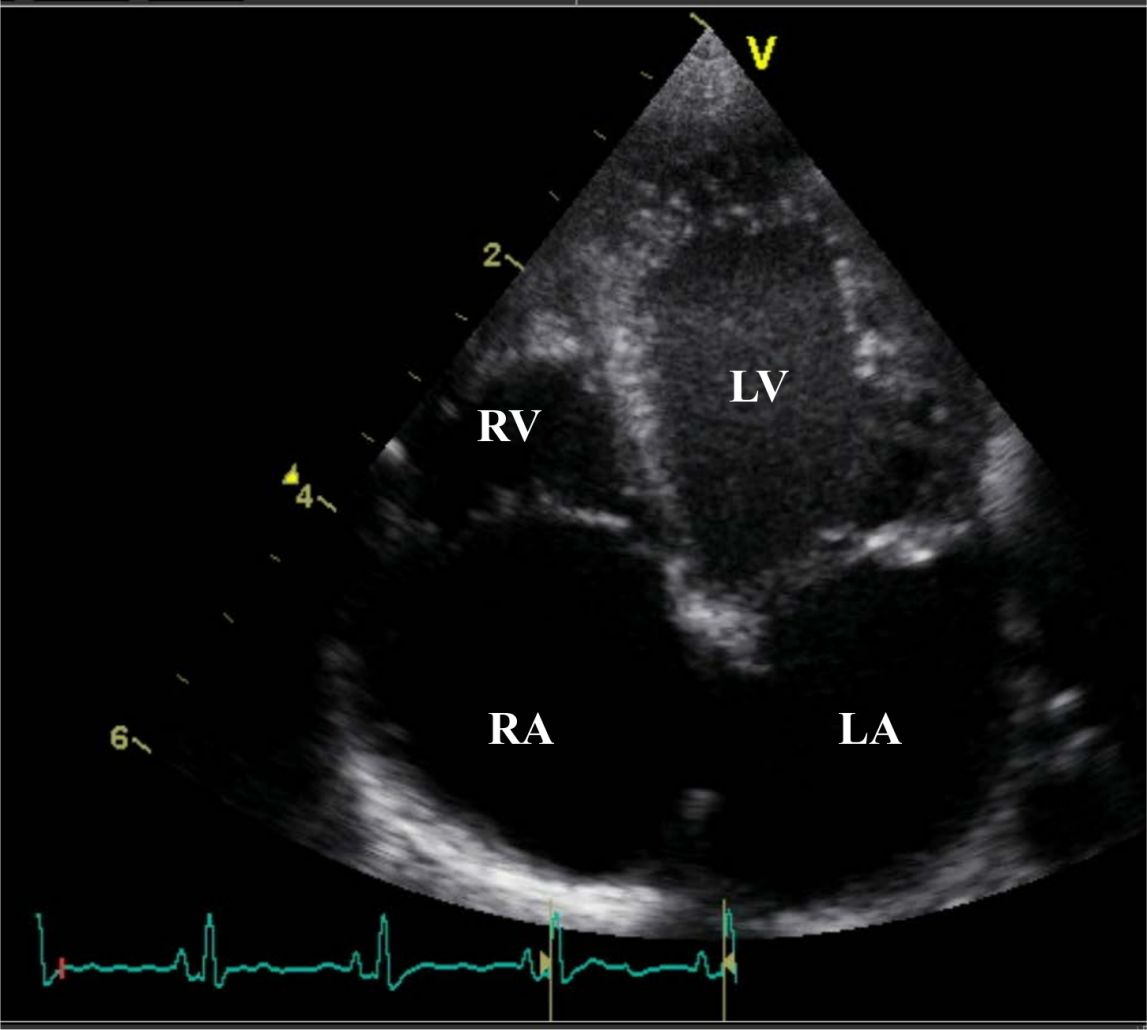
Echocardiography of index patient. Postnatal echocardiography of patient II-2 showing a bipartite RV with agenesis of the apex. Marked dilatation of the RA due to severe tricuspid insufficiency (grade 3/4). RA, right atrium; RV, right ventricle; LV, left ventricle; LA, left atrium.
